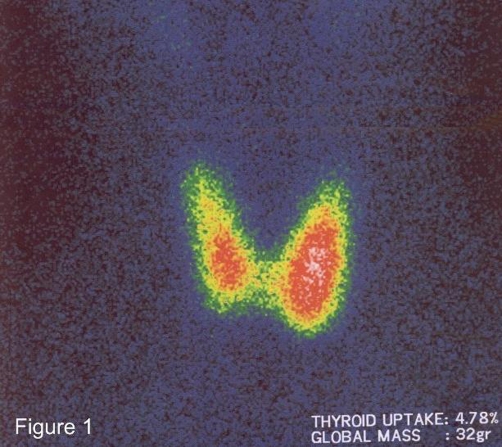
Thyroid Tc-99m scintigraphy. Diffusely increased homogenous localization of technetium pertechnetate (Tc-99m) in the thyroid gland. The gland looks enlarged, in particular the left lobe.
